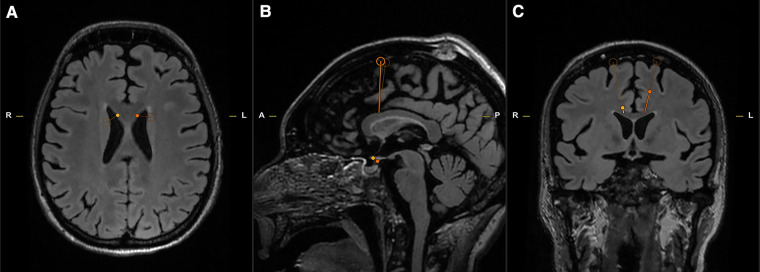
Axial (A), Sagittal (B), Coronal (C) T2 MRI slices for pre-operative stereotactic planning. The open circle indicates the point of entry, and the termination of the thin line marks the target depth (Yellow—Right; Orange – Left).
